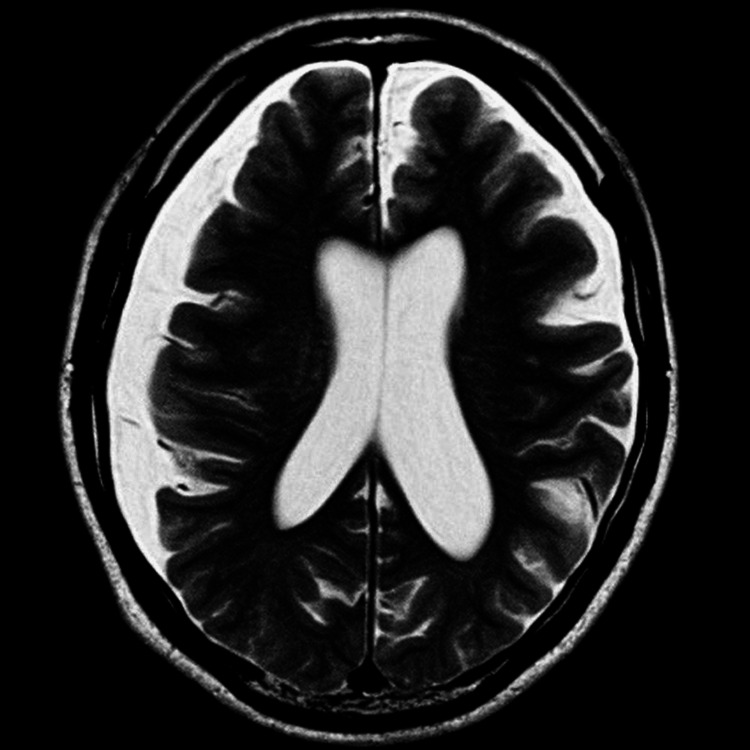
Brain MRI after the first syncopal episode. Brain magnetic resonance imaging (MRI) was performed shortly after the first syncopal episode. No evidence of acute pathology or tumor was found. Preexisting subdural effusion was noted, but no changes were observed compared to imaging performed two years prior to laryngeal cancer surgery.
